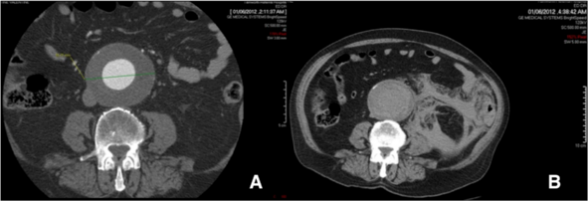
a Abdominal CT with IV contrast of a patient with symptomatic AAA. b Non-contrast abdominal CT of the same patient after collapse about 2 h later. The AAA ruptured as is evident from the retroperitoneal hematoma
